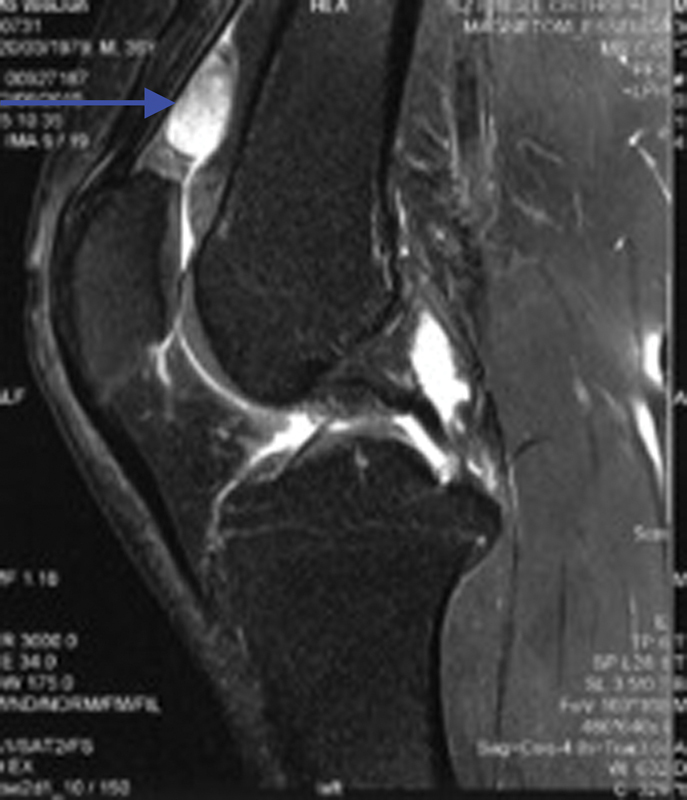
The mass was hyperintense in T2-weighted image (blue arrow).
